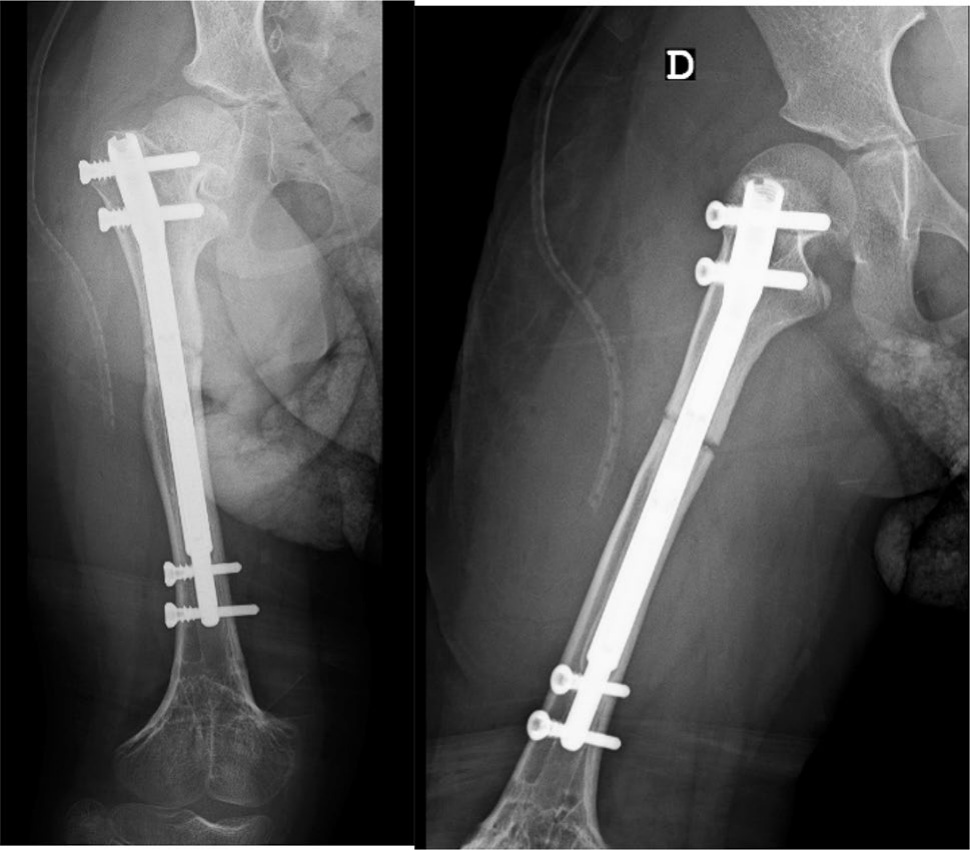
Postoperative X-ray after third surgery (new osteotomy and impute of distal locking screws)
